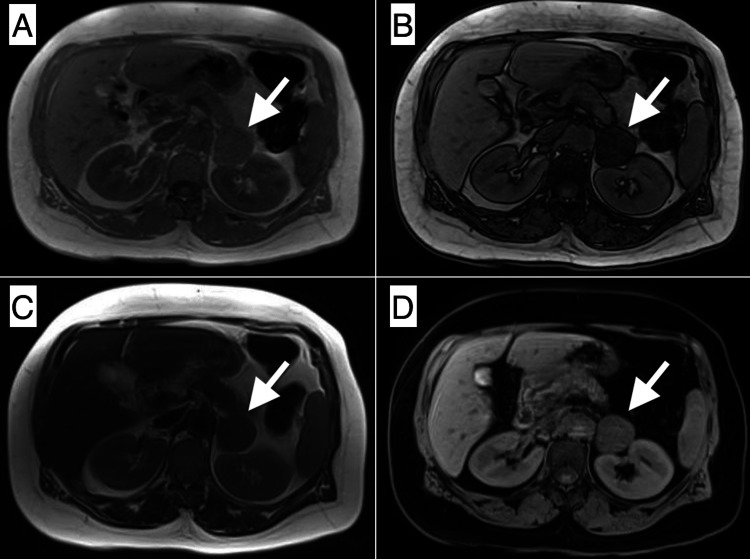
A left adrenal mass (arrow) that had the chemical shift on the in-phase (A) and out-of-phase (B) sequences and was slightly hypointense on the T2-weighted image (C) and post-contrast late-phase T1-weighted image (D) revealed.
